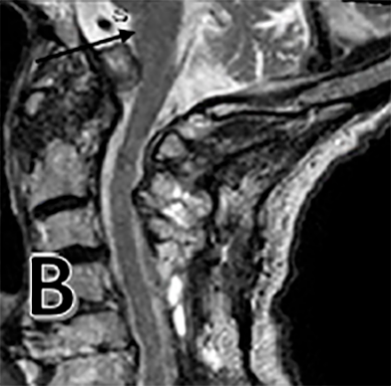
Post-operative T2 sagittal MRI showing reduction in cyst size and resolution of hyperintense signal within medulla (black arrow).
